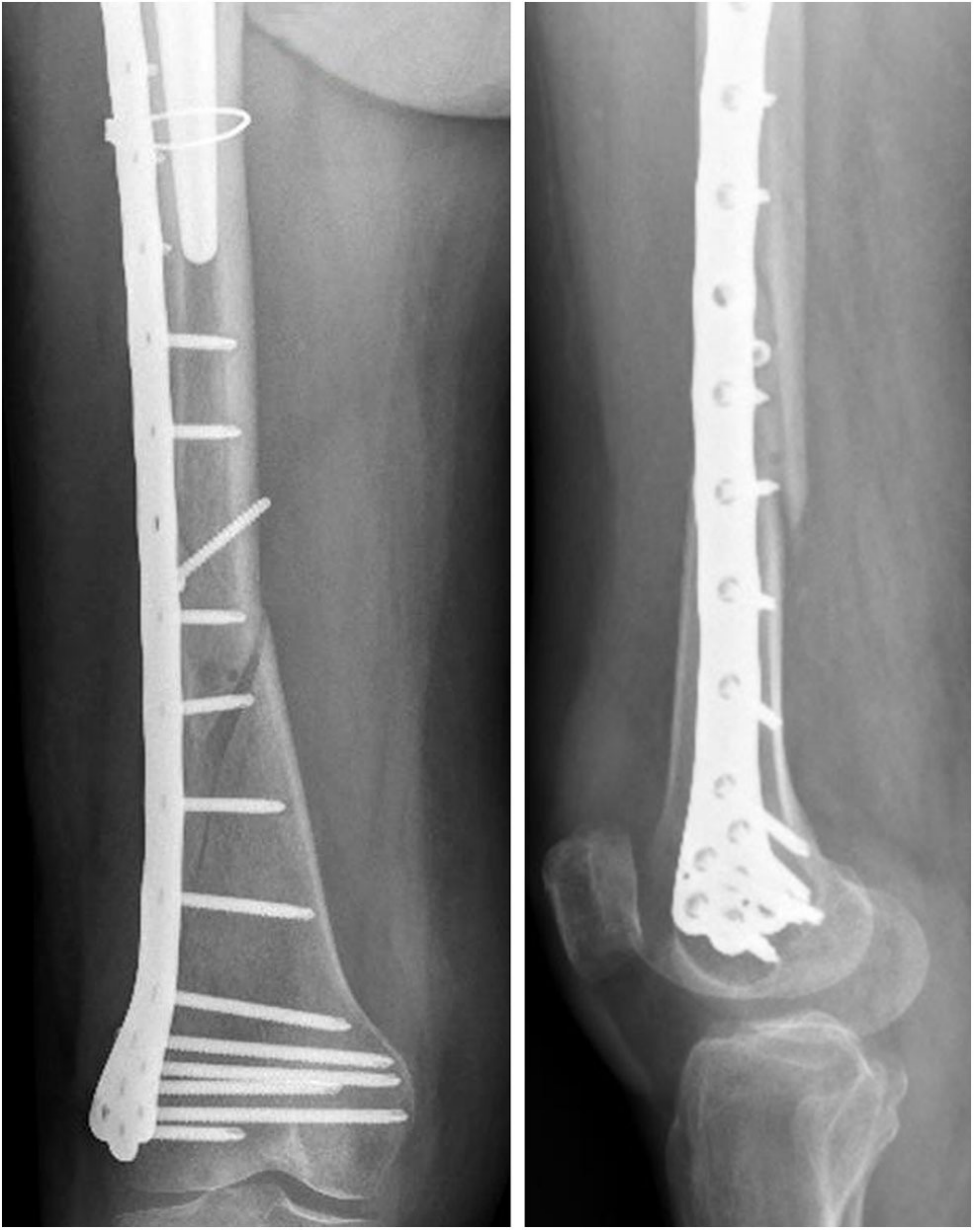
Anteroposterior (Left) and lateral (Right) x-ray images of the reduced periprosthetic fracture after the plate implant.
